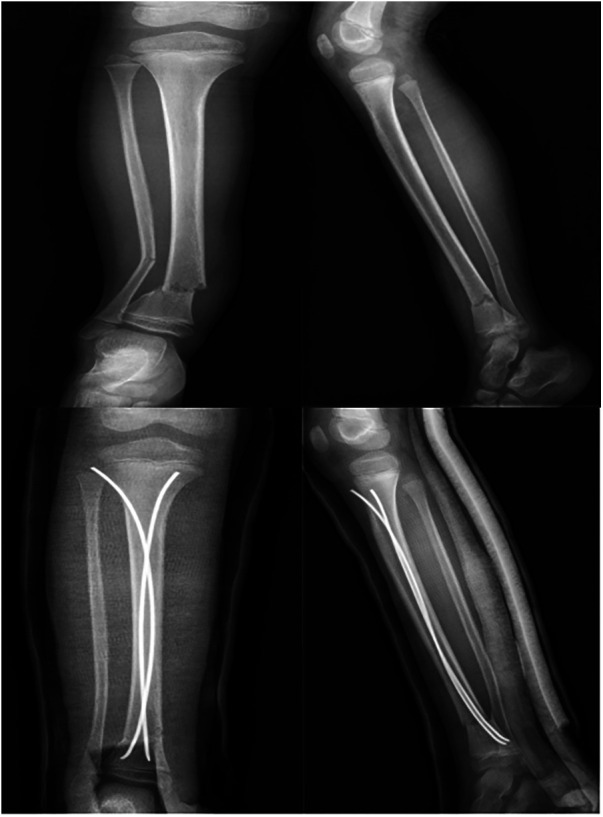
Case1: A 5-year-old boy had a fracture of the distal tibial diaphyseal metaphyseal junction(DTDMJ). X-ray films showed coronal valgus deformity of the distal tibiofibular fracture with displacement. The coronal valgus angulation remained uncorrected after treatment with the ESIN technique.
